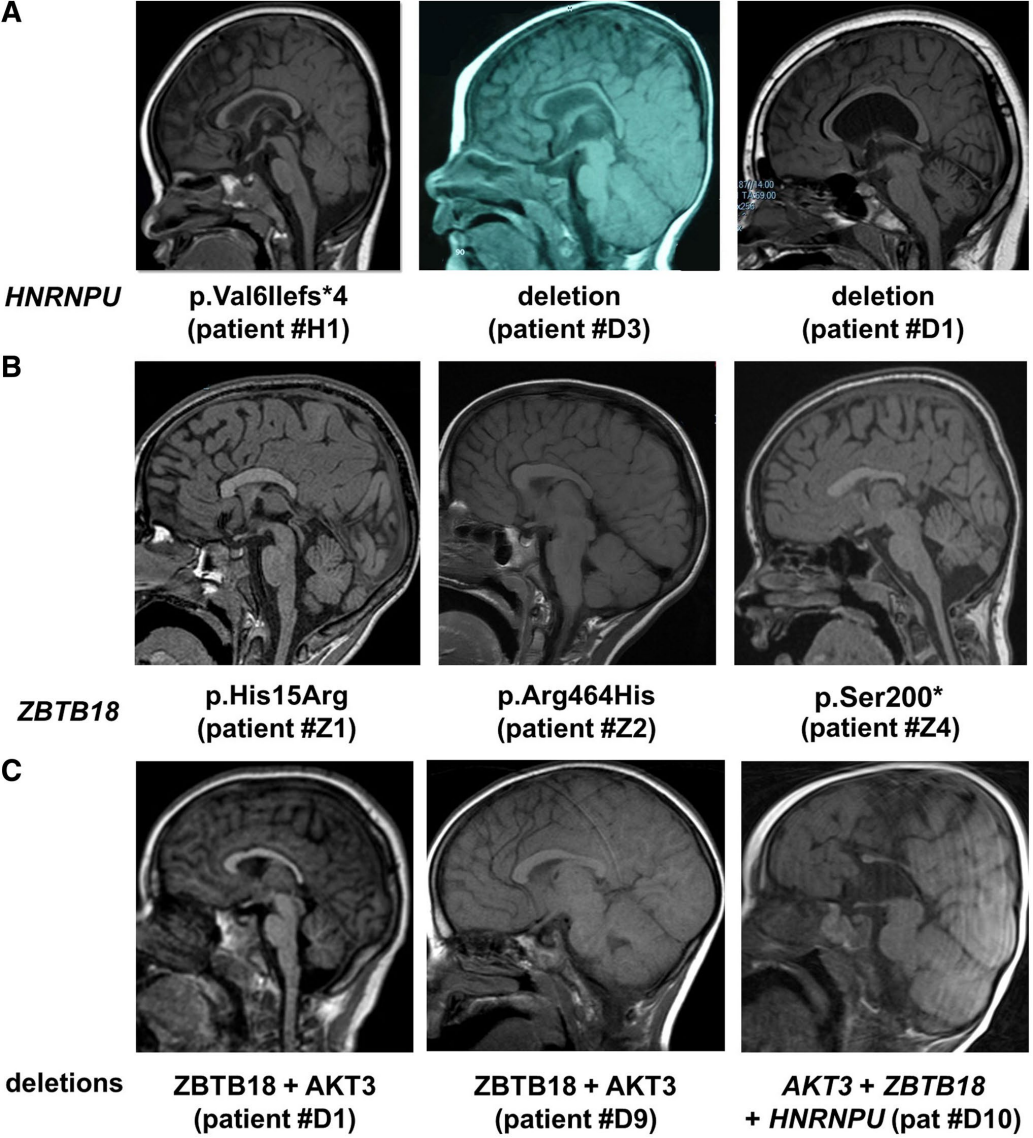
Aspects of the corpus callosum (CC) on MRI in patients with *ZBTB18* and *HNRNPU* mutations and deletions. a Normal CC in a patient with *HNRNPU* mutation (*left*) and ThCC in two patients with *HNRNPU* deletions (*middle* and *right*). b Partial AgCC (*left* and *right*) and short DysCC (*middle*) in three patients with *ZBTB18* mutations. c ThCC (*left*) and normal CC (*middle*) in two patients with *AKT3* + *ZBTB18* deletions. Partial AgCC in a patient with a deletion encompassing *AKT3* + *ZBTB18* + *HNRNPU* (*right*)
